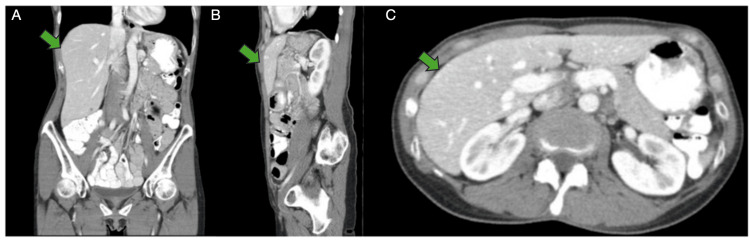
Repeat CT scan after three months in outpatient. Complete resolution of hemoperitoneum (A) Coronal view: complete resolution of hemoperitoneum (green arrow); normal abdominal viscera. (B) Sagittal view: no residual hematoma or active bleeding (green arrow); stable appearance. (C) Axial view: resolution of prior hemoperitoneum (green arrow) with normal solid organs and vasculature. CT: computed tomography
